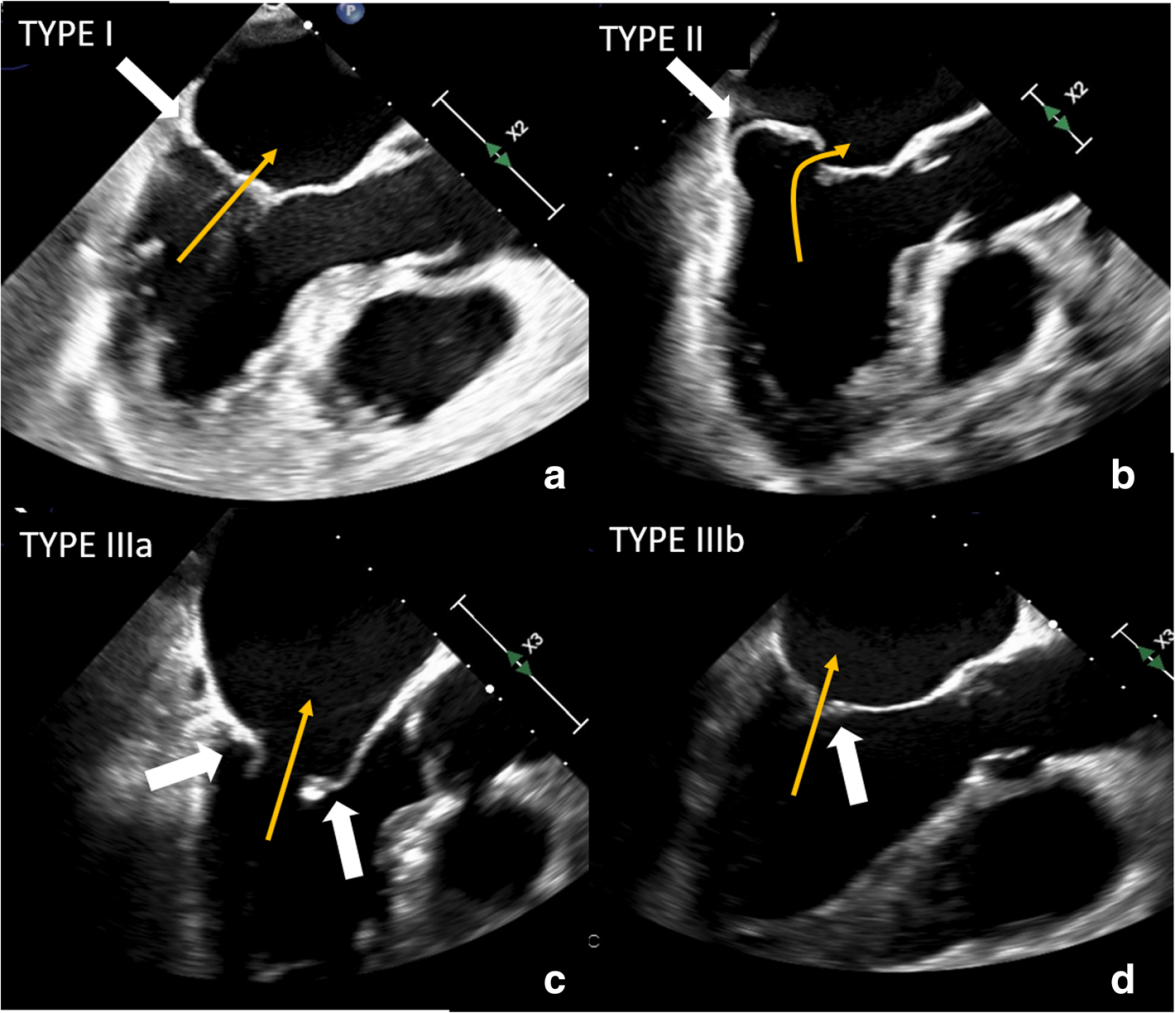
Transesophageal echocardiographic (TOE) images of mitral regurgitation (MR) according to the Carpentier classification. The MR flow direction is represented by an orange arrow. (a) An example of Carpentier type I: long-axis midesophageal (ME) view TOE showing normal valve anatomy with no prolapse or tethering but atrial dilatation/dysfunction (atrial MR) with horizontal coaptation of the leaflet (white arrow). (b) An example of Carpentier type II: prolapse of posterior leaflet (white arrow) in a valve with myxomatous degeneration (Barlow’s disease). (c) Carpentier type IIIa. Long-axis ME view TOE showing diastolic movement restriction (white arrows) in a valve affected by rheumatic disease. Of note, leaflet thickening and retraction with “hockey stick” morphology of anterior leaflet (white arrow on the right side). (d) Carpentier type IIIb. Long-axis ME view TOE showing systolic movement restriction in a patient with previous anterior myocardial infarction. Of note, the coaptation gap between mitral valve leaflets (white arrow)
